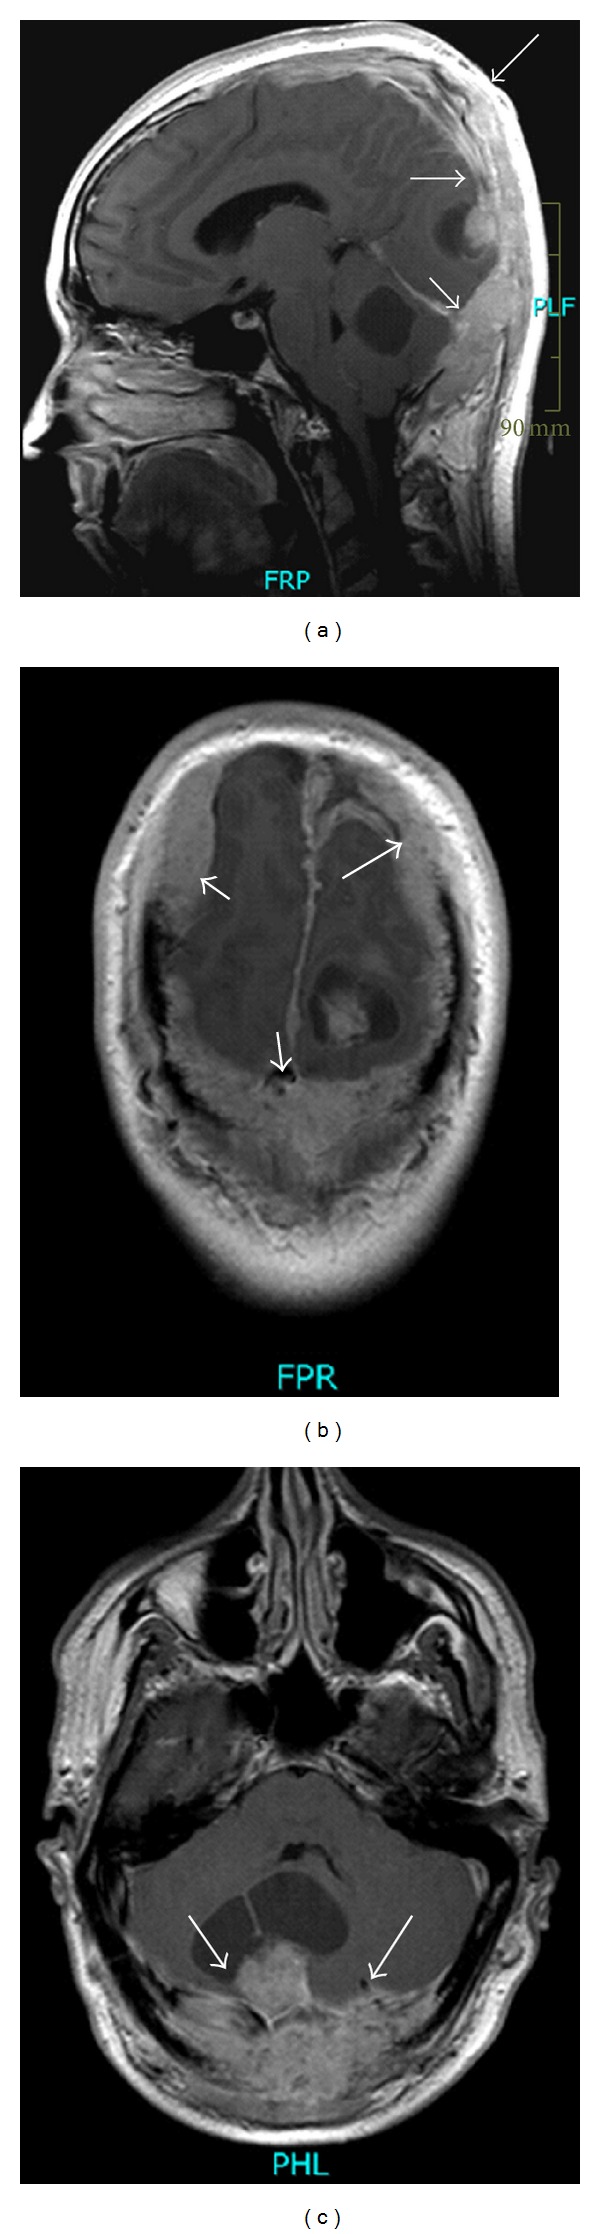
Cranial MRI with gadolinium. (a) Sagittal T1-weighted MRI demonstrates contrast enhancing tumor expanding from frontal to suboccipital. (b) Coronar T1-weighted MRI shows the fronto-parietal expansion of the tumor, cystic lesion left occipital, and the tumor mass suboccipital. (c) Axial T1-weighted indicates the tumor and cystic lesion suboccipital with compression of the forth ventricle.
